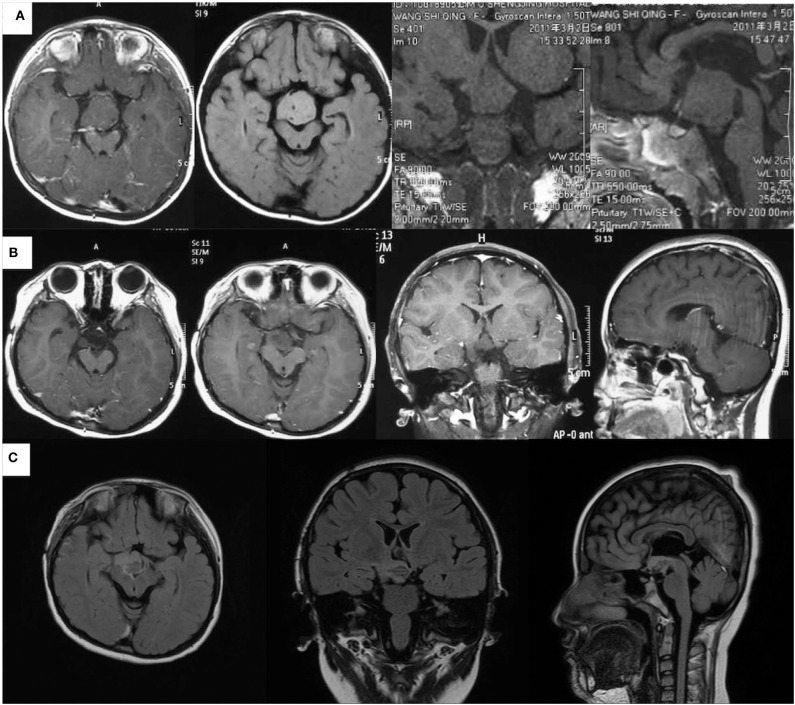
Magnetic resonance imaging scans of the patient at different ages. (A) Images at 2.5 years of age before the first resective operation the giant HH (about 35 × 32 × 25 mm, images from left to right are the axial contrasted T1 image, axial T2 FLAIRE image, coronal T1 image, and sagittal T1 image). (B) Images at 3.5 years of age after the second resective operation demonstrated about 25% of the lesion had remained (images from left to right are two axial contrasted T1 images in different slices, contrasted coronal T1 image, and contrasted sagittal T1 image). (C) Images 7 days after the ablation showed that almost all the par connection between the HH and the hypothalamus has been coagulated. (Images from left to right are axial, sagittal, and coronal T2 FLAIRE images).
